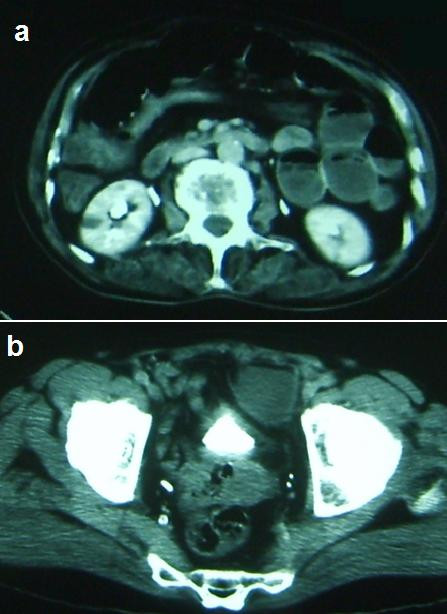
A computed tomography scan showing a distended loop with transitional zone.
